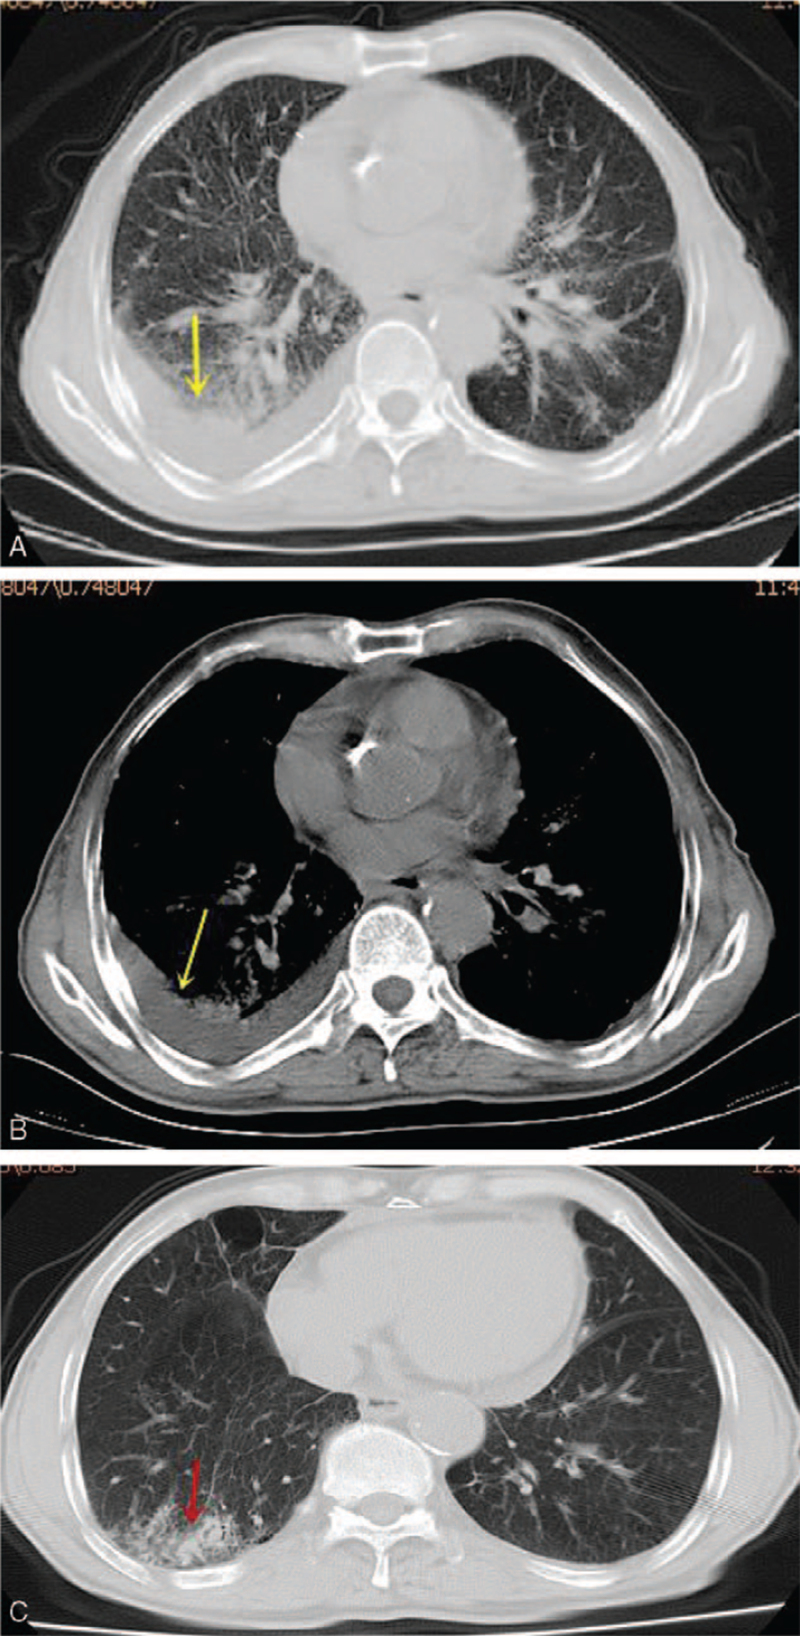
CT scan results. (A, B) The right-sided pleural effusion with adjacent pulmonary atelectasis (yellow arrow). (C) The effusion disappeared (red arrow) since the patient completed 2 cycles of chemotherapy. CT = computed tomography.
